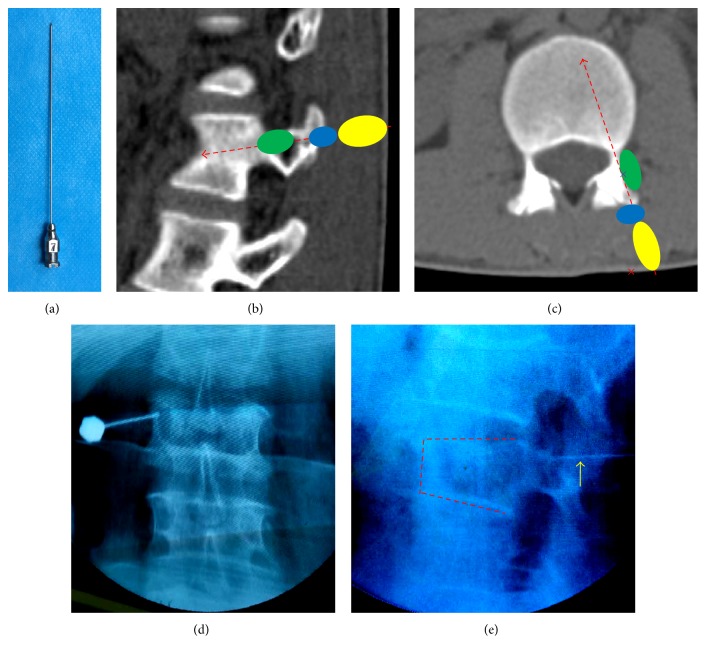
(a) Anesthetic needle (number 7 epidural needle). ((b), (c)) Red dashed arrow-puncture needle path. Yellow area-anesthetic region of the 1st step. Blue area-anesthetic region of the 2nd step. Green area-anesthetic region of the 3rd step (anesthetic region of EPIA). (c) Red ×: pedicle projection point on skin. Purple ×: anesthetic needle position in 3rd step. (d) Anesthetic needle position in 2nd step. (e) Yellow arrow-anesthetic needle position in EPIA on lateral X-ray.
